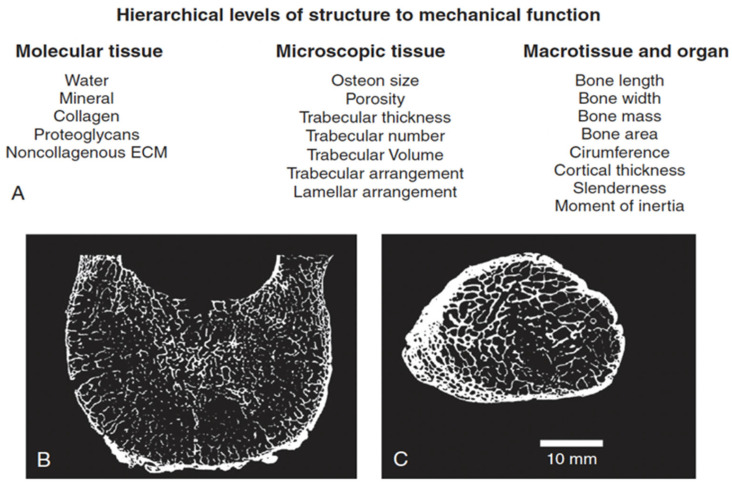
Hierarchical organization of bone. The hierarchical levels of bone composition and structure. (A) The primary component elements are at each of the three levels of structural hierarchy. The lower panel shows cross-sections (obtained via micro-computed tomography) of the human vertebra (B) and human femoral neck (C). Adapted with permission from Ref. [44]. 2021, *Wolters Kluwer Health, Inc*.
